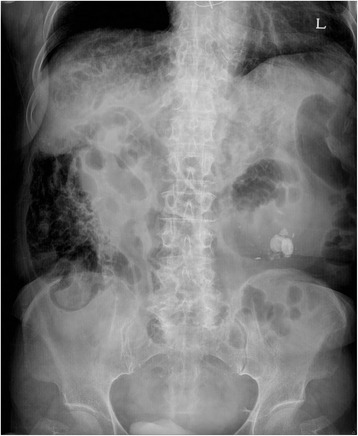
Erect view of abdominal X-ray at initial presentation
